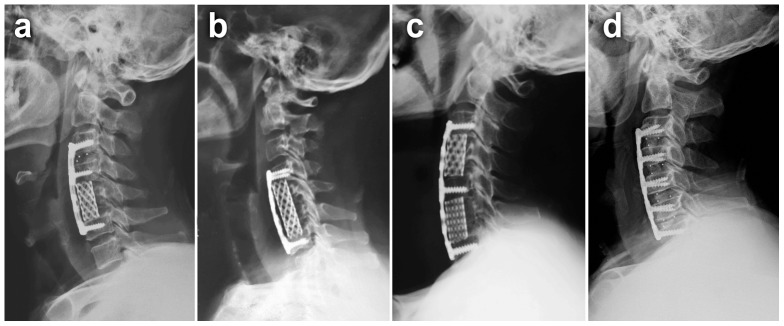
Postoperative lateral radiographs of four surgical techniques. a Anterior cervical hybrid decompression and fusion (ACHDF). b Anterior cervical corpectomy and fusion (ACCF). c Discontinuous corpectomy and fusion (DCF) with reservation of the middle vertebra. d Anterior cervical discectomy and fusion (ACDF).
